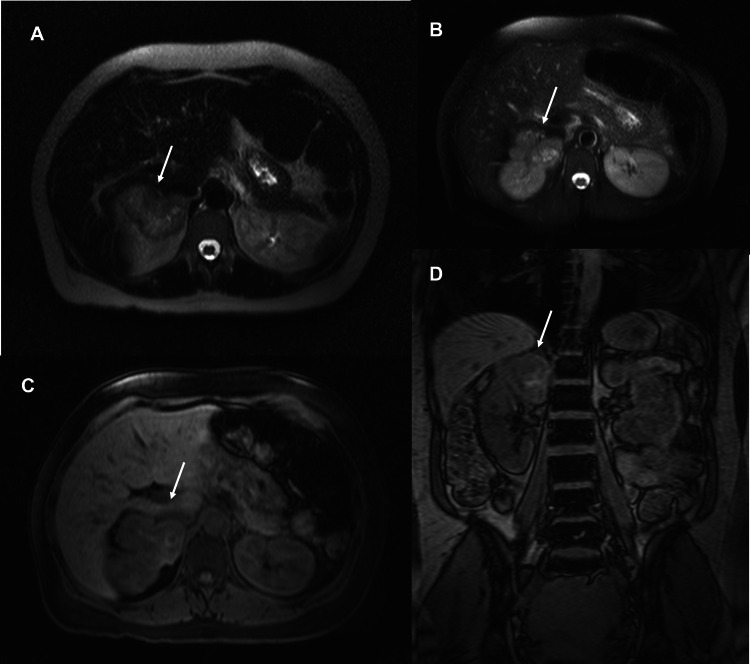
Abdominal MRI. An irregular and lobulated tumor lesion is observed in abdominal MRI images (A, B, C, D), poorly defined, adjacent to the upper pole of the right kidney: adrenal mass (arrow), from which it is inseparable, and it extends into the medial aspect of the kidney, reaching the renal hilum, along the path of the inferior vena cava, highly suggestive of pheochromocytoma.
